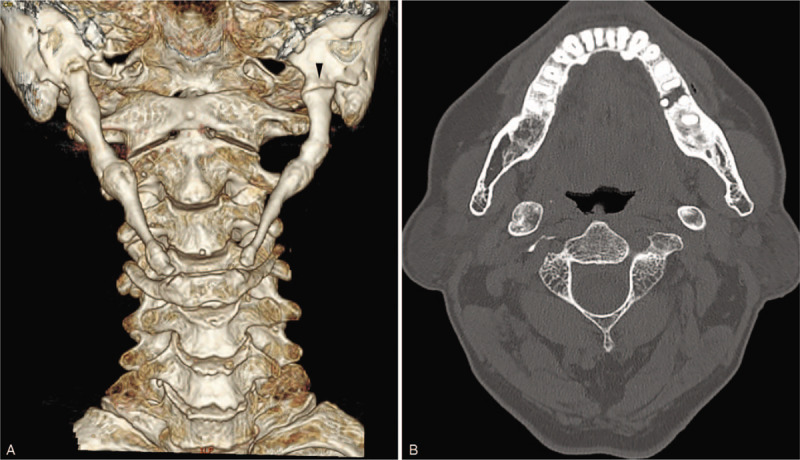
(A and B) Computed tomography scans show complete union at 1 year after trauma. (A) Note the gap on the left side appeared unchanged (arrowhead).
